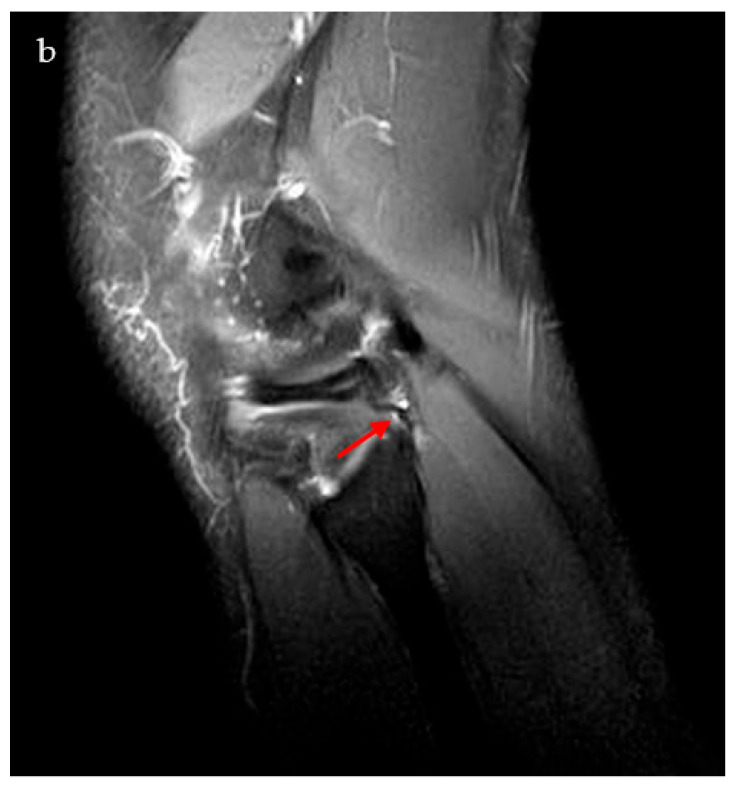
(a) Sagittal proton density-weighted (PDw) magnetic resonance image (1.5 T) demonstrating the anteroinferior meniscopopliteal fascicle (aiPMF) (white arrows), which courses obliquely from the posterior horn of the lateral meniscus toward the popliteus tendon, contributing to stabilization of the popliteomeniscal complex. The aiPMF is often thin and may not be consistently identifiable on routine MRI examinations. (b) Sagittal PDw MRI showing rupture of the anteroinferior meniscopopliteal fascicle, which appears elongated and lax (red arrow), associated with a concomitant tear of the posterior horn of the lateral meniscus.
